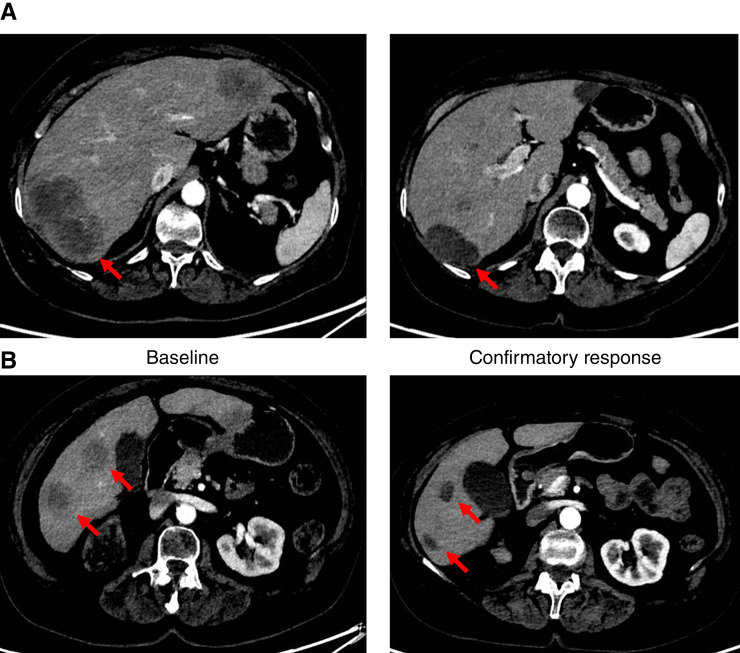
Dabogratinib demonstrates early clinical activity in a patient with mUC with an FGFR3::TACC3 fusion. CT images of a target lesion in the liver (A) and nontarget lesions in the liver (B) are shown at baseline and at 15.4 weeks as a confirmatory PR.
